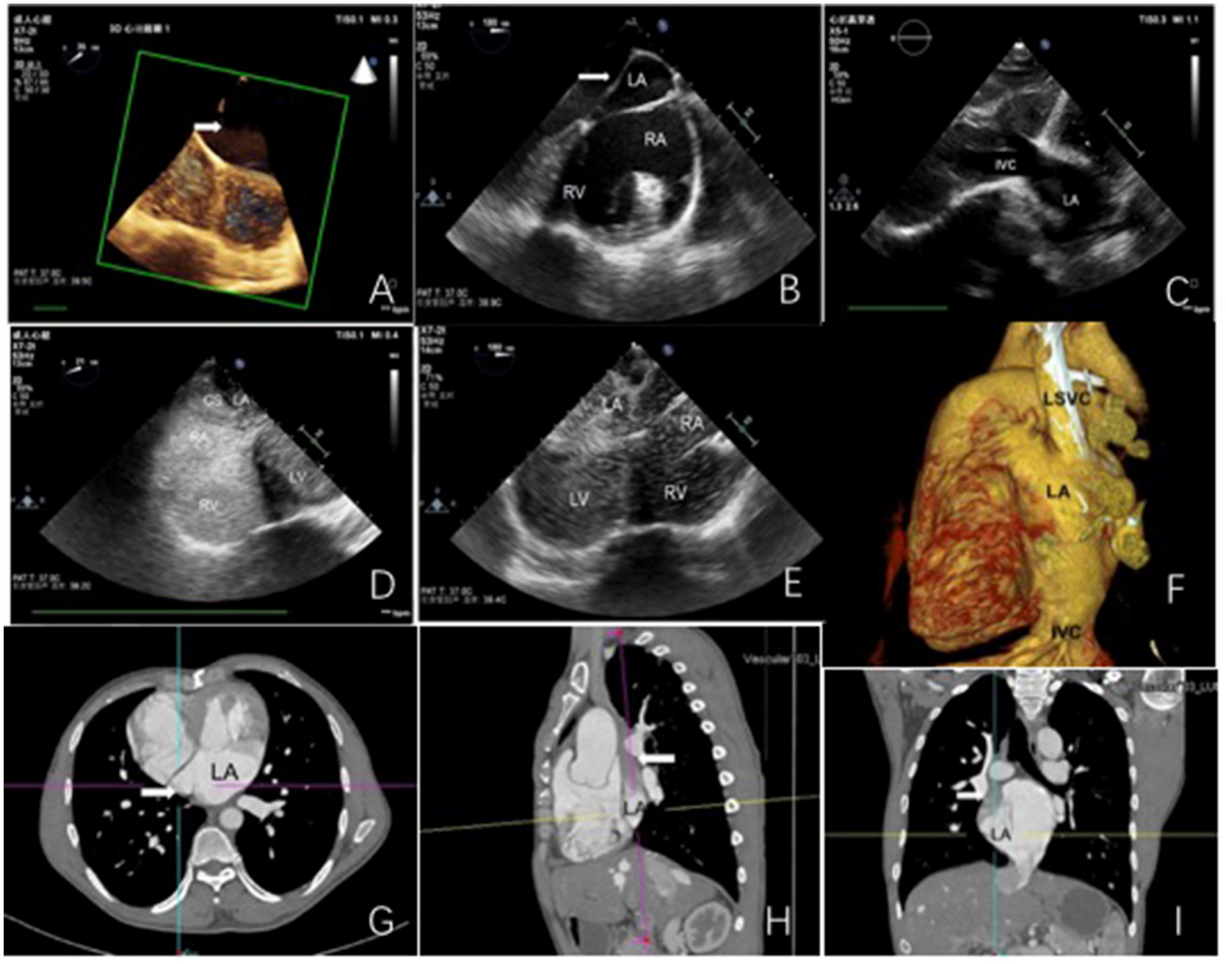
Echocardiography and computed tomography angiography. (A) complete coronary sinus atrial septal defect (white arrowheads). (B) white arrowheads show an abnormal conduit. (C) A connection between the inferior vena cava and the left atrium. (D) saline microbubbles injected into the lower limb vein expressed first in the LA and left ventricle (LV), and then in the RA and RV. (E) saline microbubbles injected into the right elbow vein expressed almost simultaneously in the LA and RA. (F) CT shows the left superior vena cava and the inferior vena cava draining into the LA. (G–I) CT shows the superior vena cava (white arrowheads) draining into the.
